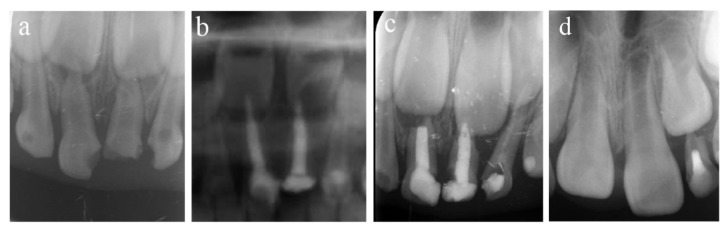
#51 and #61 were treated with pulpectomies under DGA with a 51-month follow-up period (52-month-old male child). (a) The radiograph before treatment and #51 revealed periapical radiolucency. (b) One week later, the postoperative radiograph showed optimal filling in both teeth. (c) Twenty-two months after the treatment, radiography showed physiological root resorption. (d) Fifty-one months after treatment, the permanent upper incisors erupted normally. All pulpectomies succeeded.
